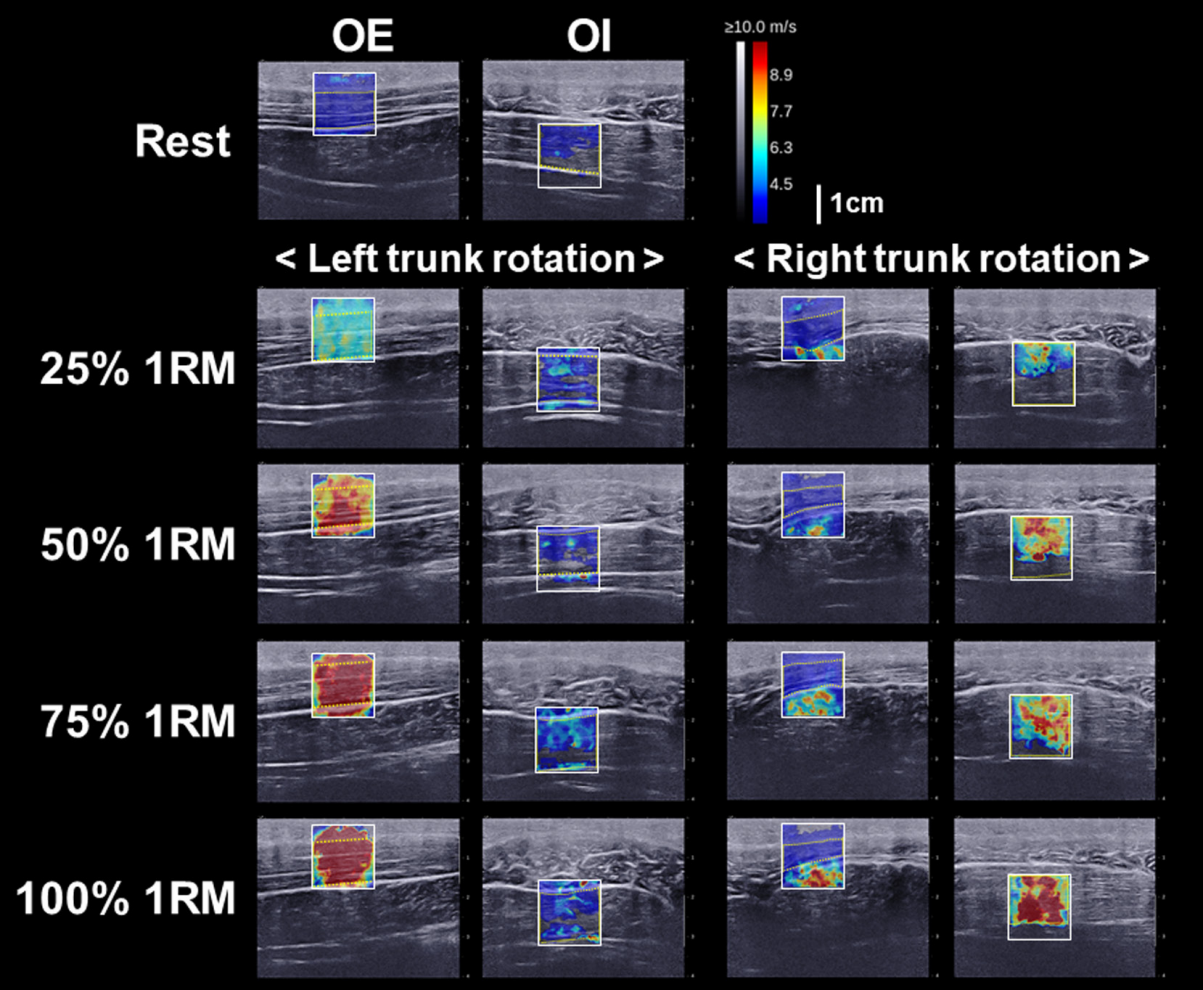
Typical color‐coded elasticity maps of the right oblique externus abdominis (OE) and right oblique internus abdominis (OI) during left and right trunk rotations. The elasticity maps were acquired using ultrasound shear wave elastography (SWE) at rest and during left and right trunk rotations at 25%, 50%, 75%, and 100% of the one‐repetition maximum (1RM). The 1.5 × 1.5 cm white squares indicate the region of interest for ultrasound SWE, and the yellow dotted line indicates the area where the average shear wave velocity of the OE and OI was quantified
